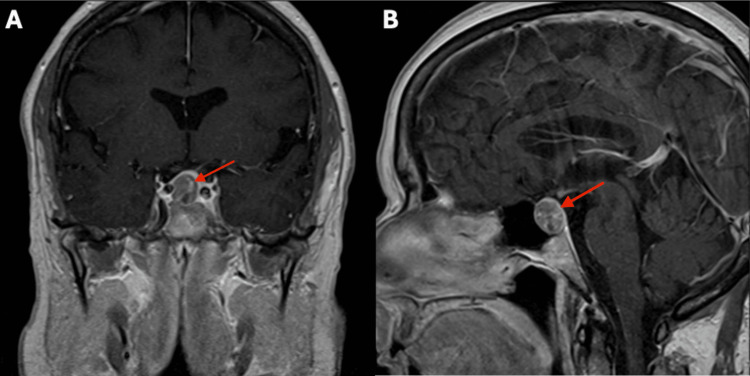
Coronal (A) and sagittal (B) T1-weighted MRIs showing NF-PitNET prior to resection The red arrow indicates the NF-PitNET, measuring 1.2 cm × 1.5 cm. NF-PitNET, nonfunctioning pituitary neuroendocrine tumor
